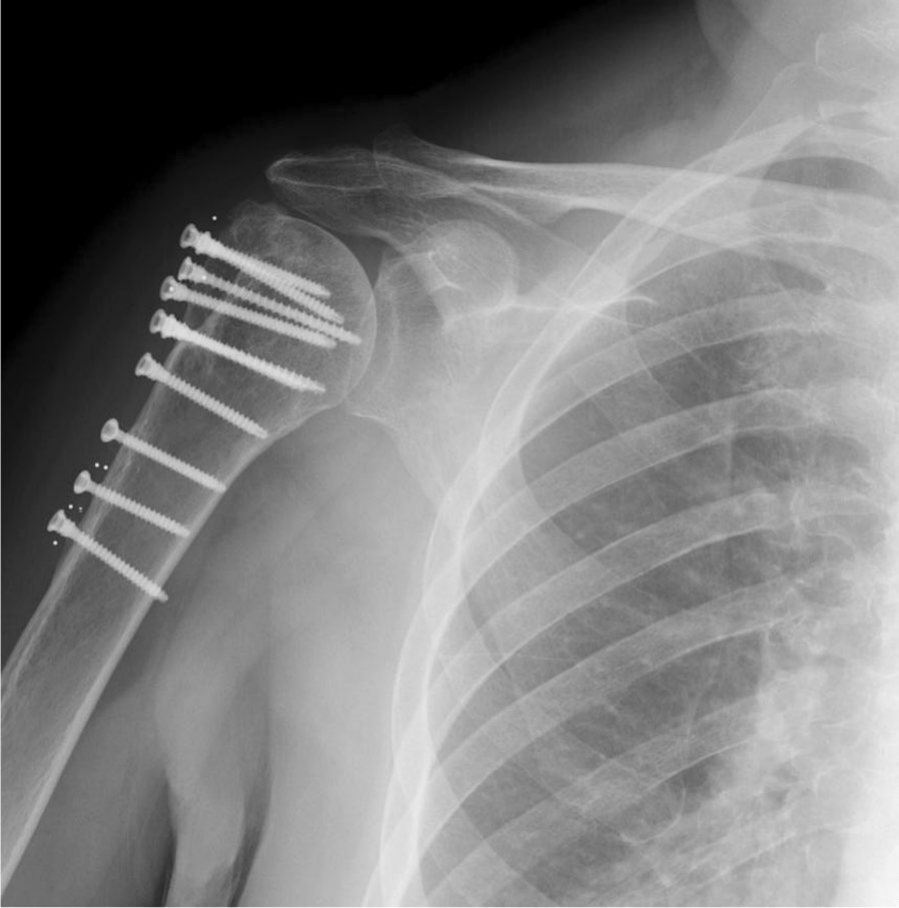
Postoperative X-rays after plate fixation
